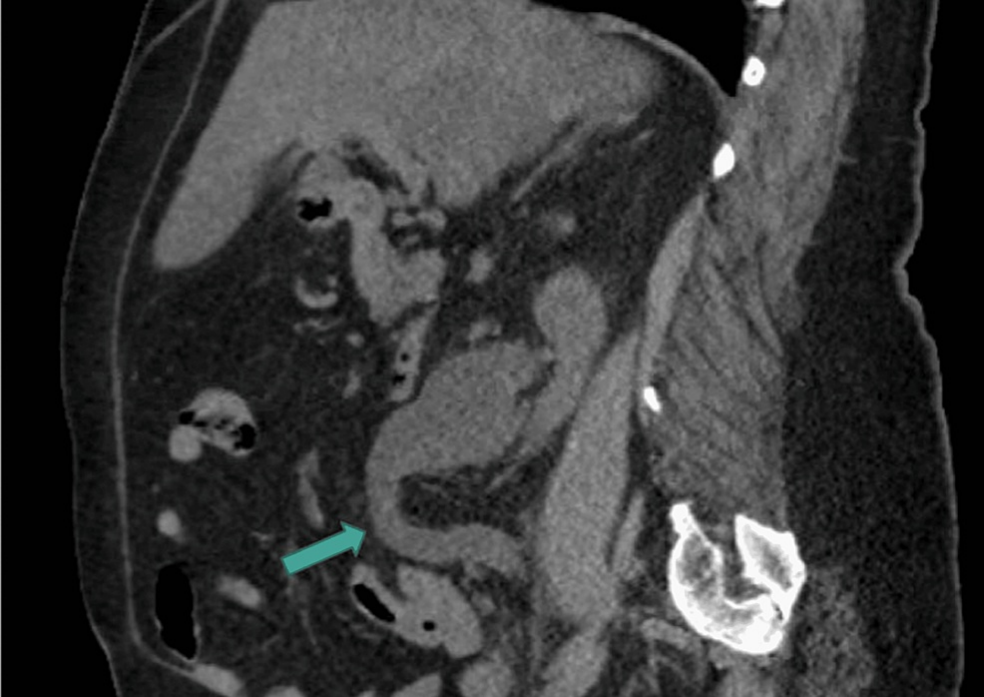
CT sagittal view revealing hydronephroureter CT: computed tomography
